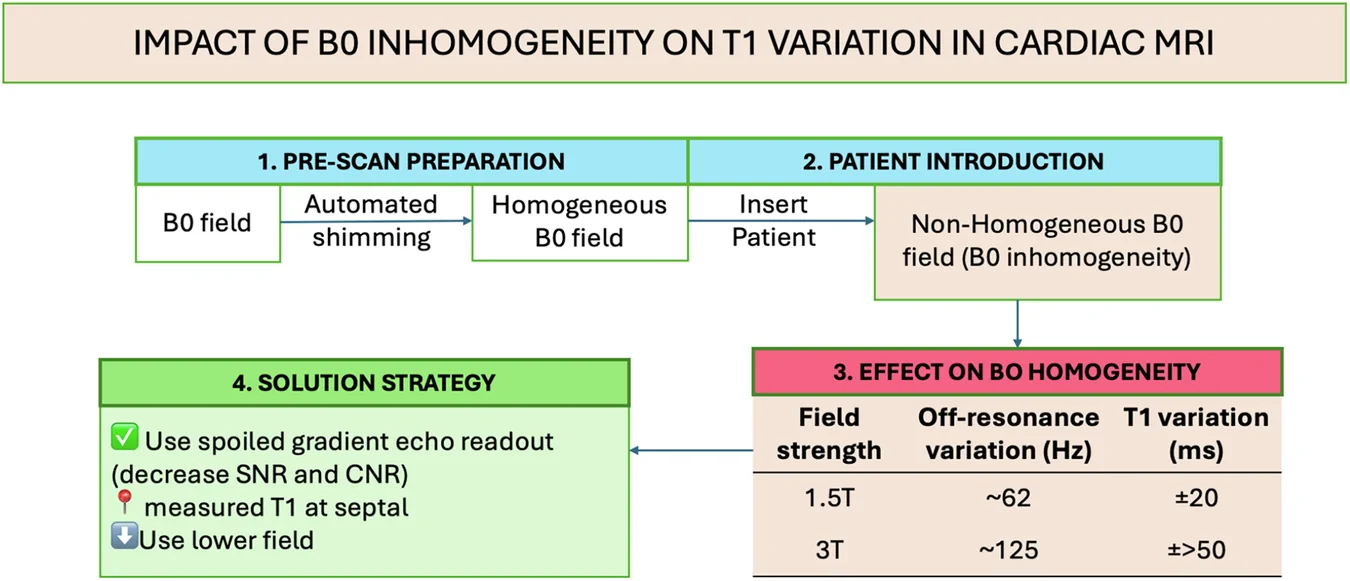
Impact of B0 inhomogeneity on T1 variation in cardiac MRI
